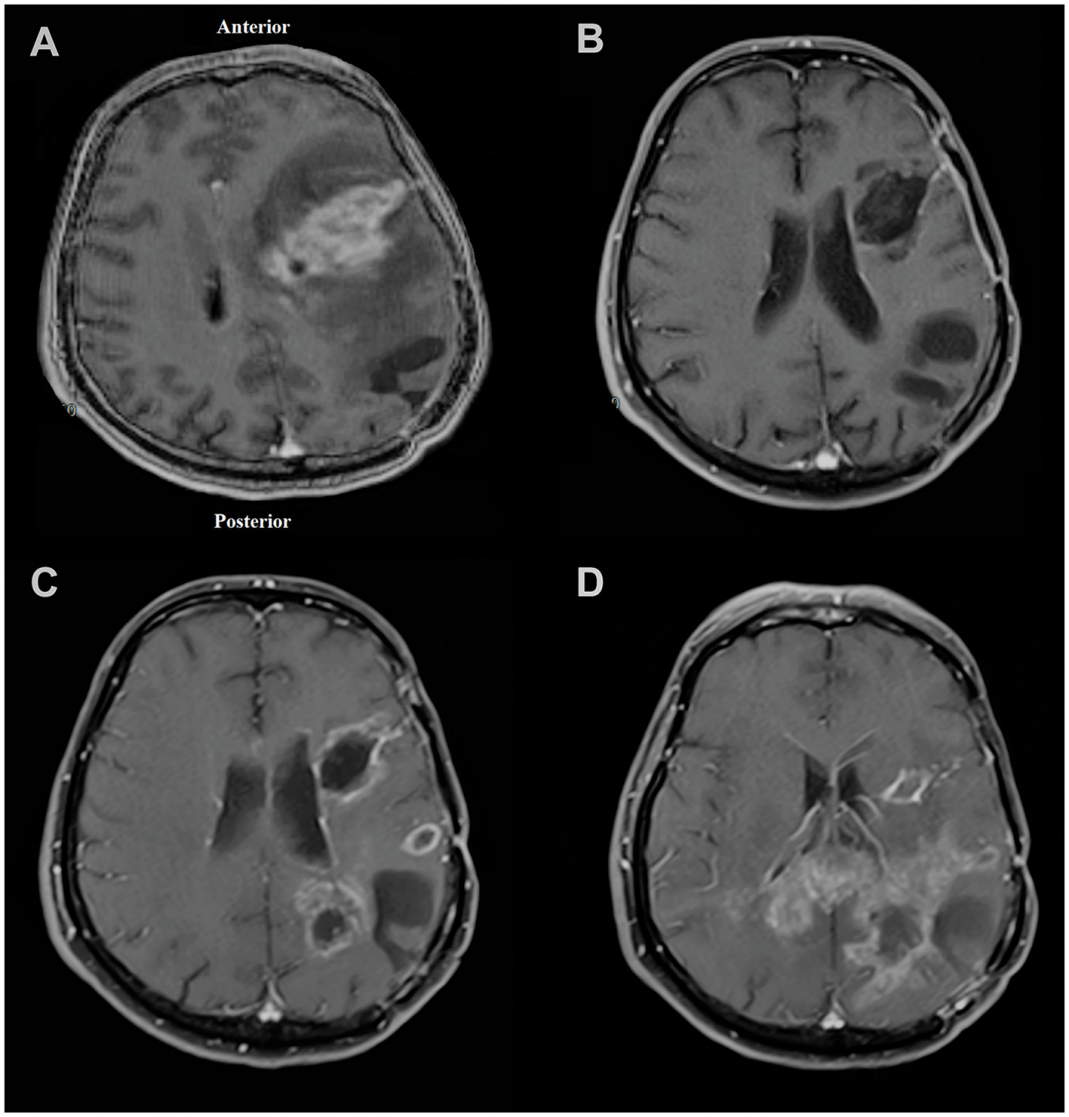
Magnetic resonance imaging of the patient in (A) November 2018 showing left frontal lesion and evidence of craniotomy related to previous surgery for parietal lesion 1 month earlier; (B) February 2019 with no obvious evidence of recurrence at the time of injection to the frontal focus; (C) December 2019 showing first evidence of recurrence of tumor mostly at the parietal site; (D) March 2020 showing left frontal focus had no prominent recurrence; however, the size of the left parietal focus increased and extended to the contralateral hemisphere through the corpus callosum.
